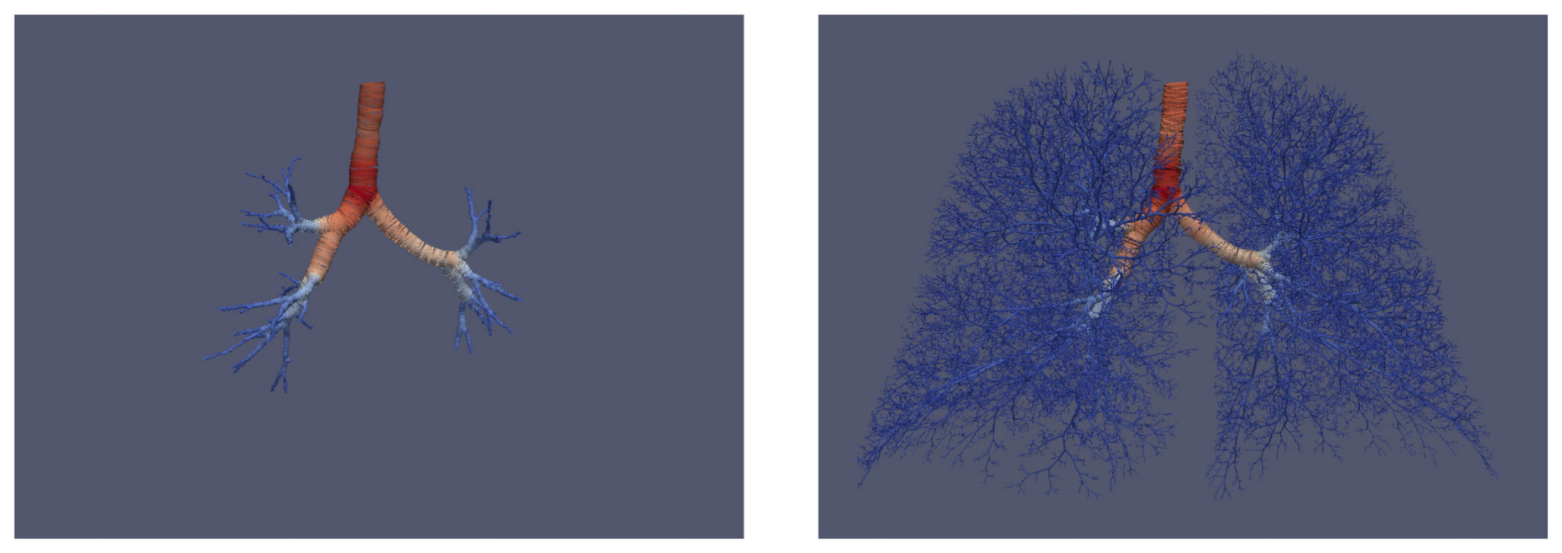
The initial geometry of major airways segmented from a CT scan (left), and an example of a complete generated airway tree (right).
